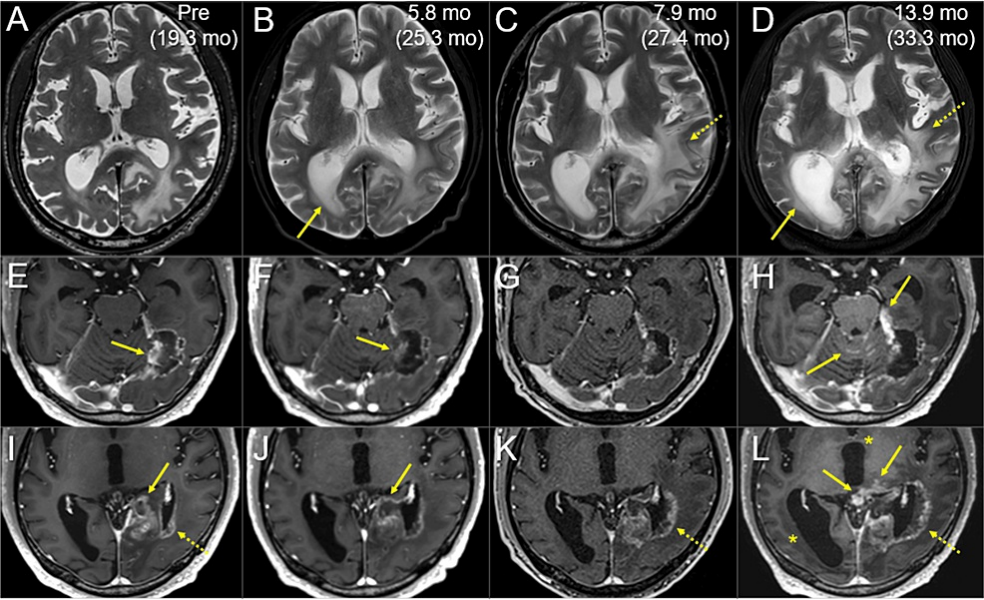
Magnetic resonance images before and after the re-radiosurgery, focusing on the progressions of radiation injury and tumor dissemination The images show (A-D) axial T2-WIs; (E-L) axial CE T1-WIs; (A, E, I) five days before the re-SRS (19.3 months after the initial SRS); (B, F, J) at 5.8 months after the re-SRS initiation (25.3 months after the initial SRS); (C, G, K) at 7.9 months (27.4 months) and (D, H, L) at 13.9 months (33.3 months). (A-L) These images are shown at the same magnification and coordinates under co-registration and fusion based on the pre-SRS images. The image series of A-D, E-H, and I-L each show different cross-sections. (A-D) The contralateral lateral ventricle dilated (arrow in B) at 5.8 months and progressed (arrow in D), along with aggravation of the perilesional/paraventricular edemas (dashed arrows in C, D). (E-L) Although the regrowth lesions almost regressed (arrows in E, F, I, J), meningeal dissemination appeared on the cerebellar surface and around the brainstem, along with the increased enhancement of the tentorial incisura (arrows in H, L). Brain radionecrosis around the left lateral ventricles progressed (dashed arrows in I, K, L), along with progression of triventriculomegaly (asterisks in L). WI: weighted image; CE: contrast-enhanced; SRS: stereotactic radiosurgery; mo: months
